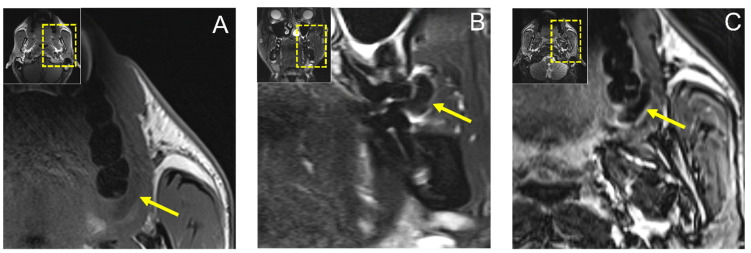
(A) Axial reconstruction of native T1 and (B) coronal reconstruction of T2 fat-saturated (FS) Turbo Spin-Echo (TSE) MRI protocols showing a hypointense lesion in T1-weighted images in axial orientation measuring approximately 20 × 5 mm and a 12 × 8 mm hypointense lesion in coronal T2 FS TSE reconstruction, ruling out a fat-containing tumor or cystic process. In addition, (C) axial T2-weighted Dixon TSE reconstruction is shown.
